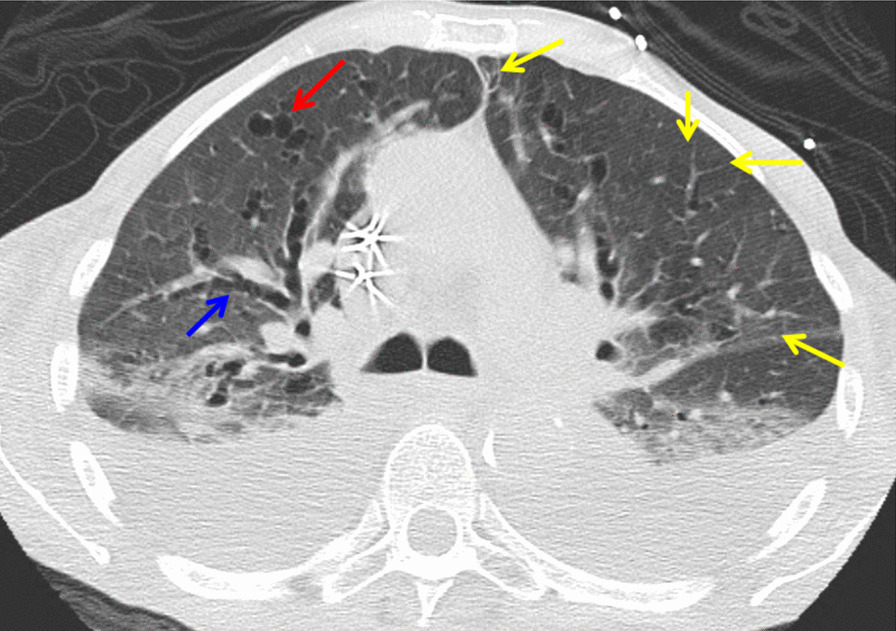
High-resolution computed tomography of the chest shows severe bronchiectasis (blue) bilaterally with septal thickening (yellow), and thin-walled cysts (red) consistent with radiographic features of lymphocytic interstitial pneumonia, a well-described interstitial lung disease in pSS. Bilateral pleural effusion was notable
